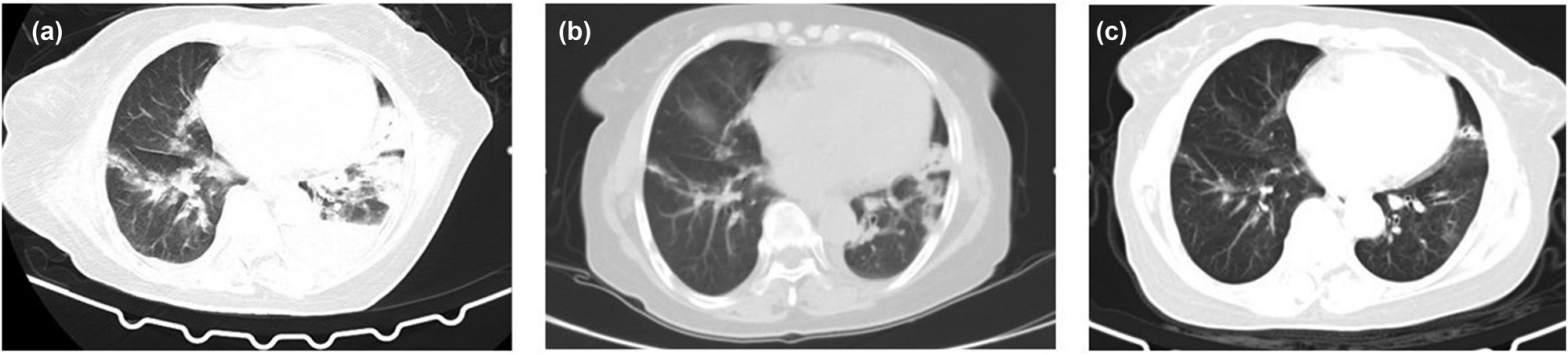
The CT image of case 1. Picture (a) (2020.10.19) is admission D12 and DOX D4 is used, showing multiple patchy and nodular increased density shadows in both lungs, the left lung is obvious, and the left pleural effusion. Picture (b) (2020.10.28) shows admission D22, DOX D14, and the lesions in both lungs were obviously absorbed. Picture (c) (2020.12.07) was reviewed 1 month after discharge, and the lesions in both lungs were completely absorbed.
